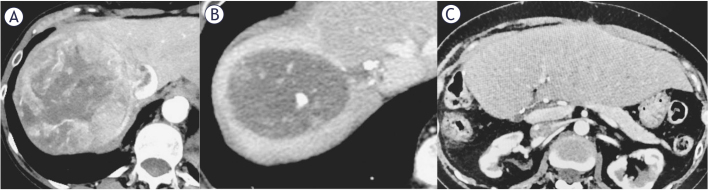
58-year-old female with a 116 mm hepatocellular carcinoma (HCC) in the right hepatic lobe, with a tumor thrombus (Japanese classification Vv3) in hepatic vein and inferior vena cava. (A). Follow up CT scan after 8 months demonstrated a complete response to transarterial radioembolization (TARE) and atezolizumab/bevacizumab treatment (B). Last follow-up CT scan was 7 months after surgery and showed no signs of disease progression (C).
